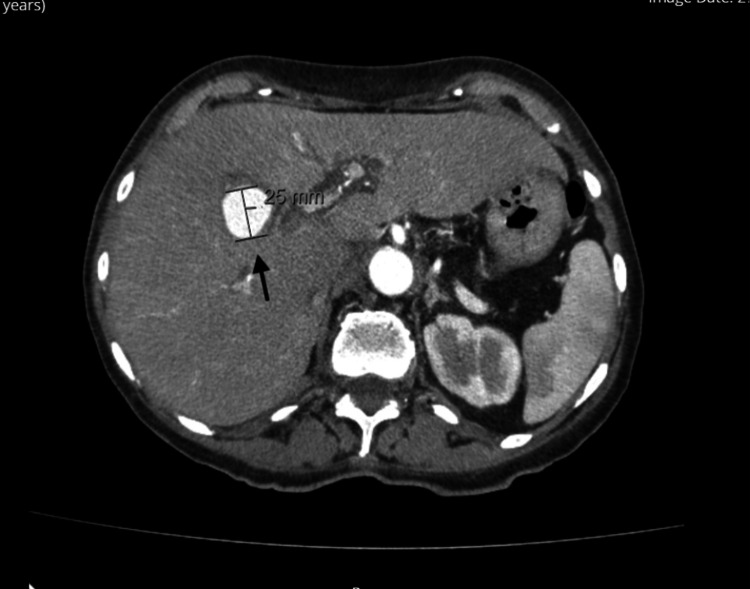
Triple phasic Computed Tomography of the liver in the axial view demonstrating a 3 cm x 2.5 cm aneurysm (black arrow) arising from a branch of the left hepatic artery.
